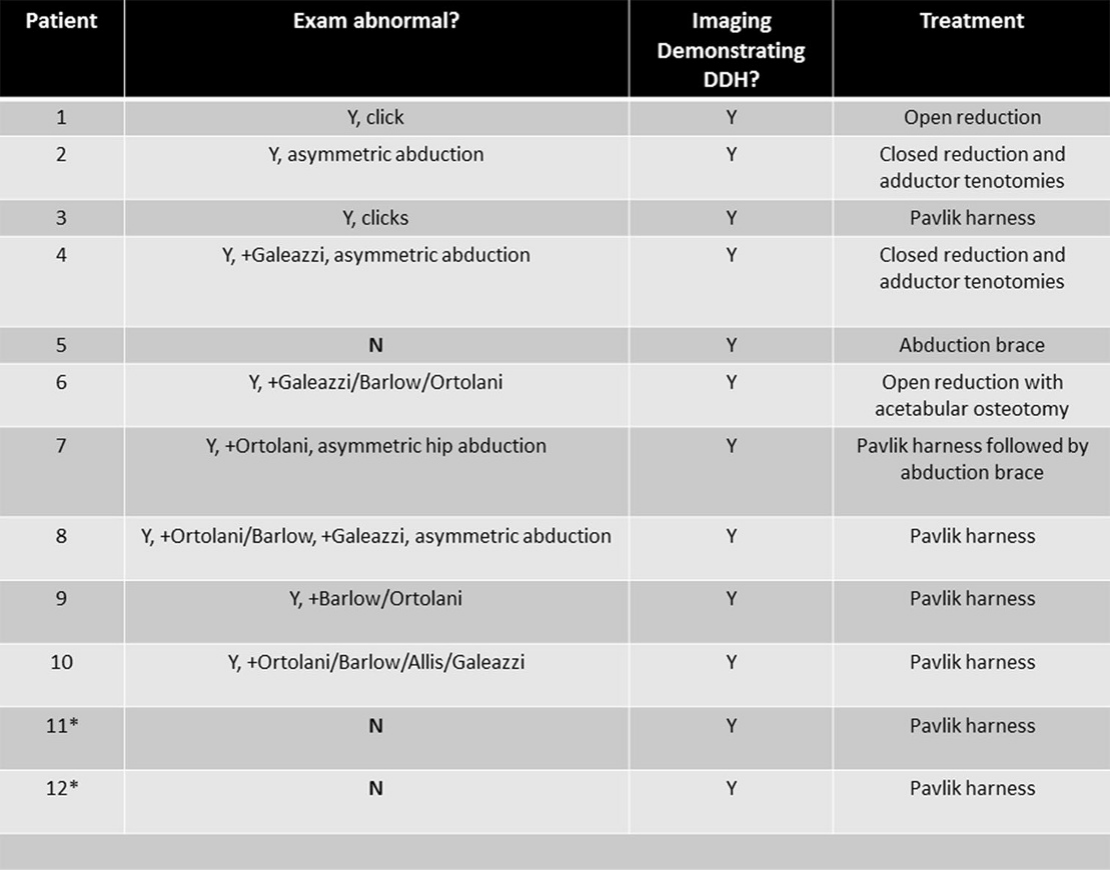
Patients with DDH demonstrating (1) physical exam abnormalities, (2) radiographic abnormalities, and (3) treatment received. *Presented as second opinion, previously treated. Used with the permission of the Children’s Orthopaedic Center, Los Angeles
